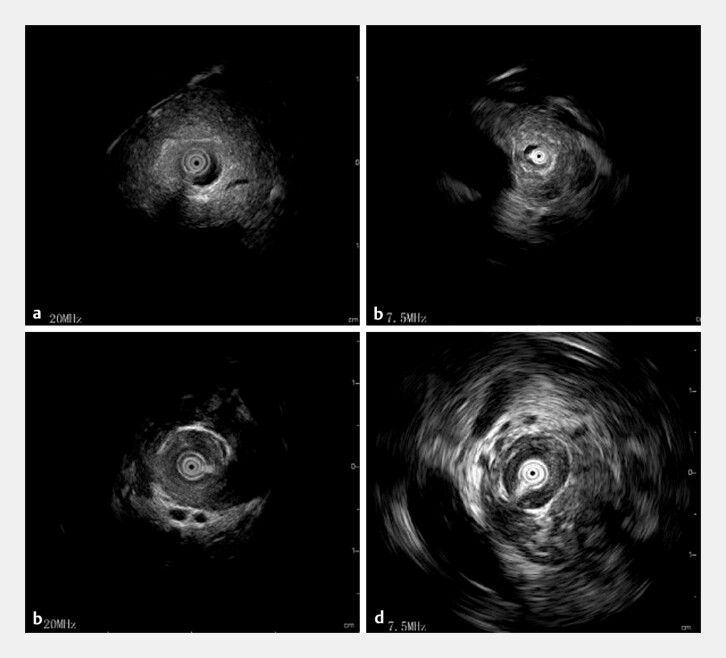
Images during dual-frequency intraductal ultrasonography (IDUS) showing;
